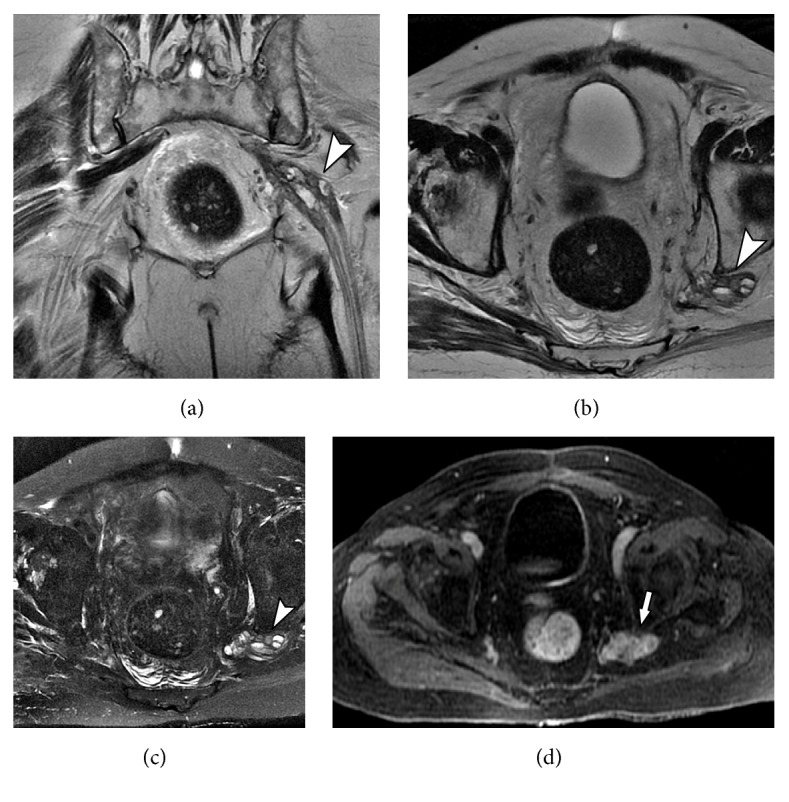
73-years old male with history of weakness and paresthesia in the lower left limb. Previous history of left nephrectomy due to renal tumor 5 years ago. MRN images on coronal T2 3D isotropic (a), axial T2 (b), axial T2 with fat suppression (c) and axial axial T1-weighted contrast-enhanced (d) showing a solid tissue and neural thickening of the proximal sciatic plexus, with contrast enhancement (solid arrow) and cystic areas of permeation (arrowhead), characterizing neoplastic infiltration.
